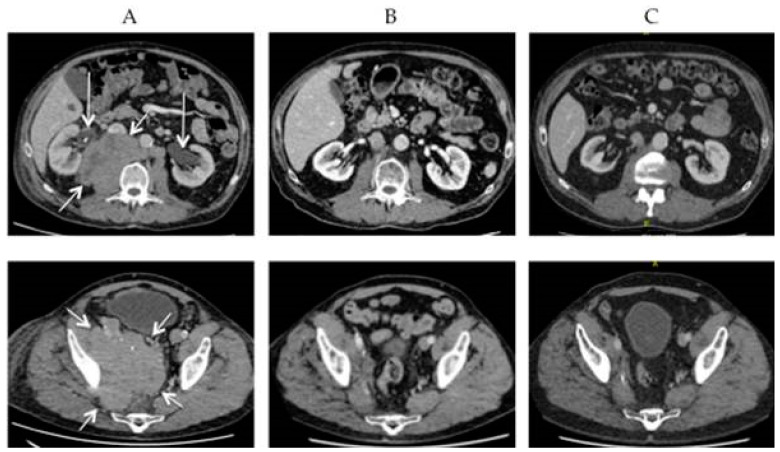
CT-scans of a 66-year-old man with massive lymphogenic metastases in abdomen and pelvis (short arrows) progressive under avelumab. Subsequent treatment with radiotherapy and restart of avelumab in last week of radiotherapy. (A) Before radiotherapy, note bilateral hydronephrosis (long arrows); (B) two months after radiotherapy (13 × 3 Gy), complete regression and recovery of hydronephrosis; (C) two years after radiotherapy; persistent complete regression.
